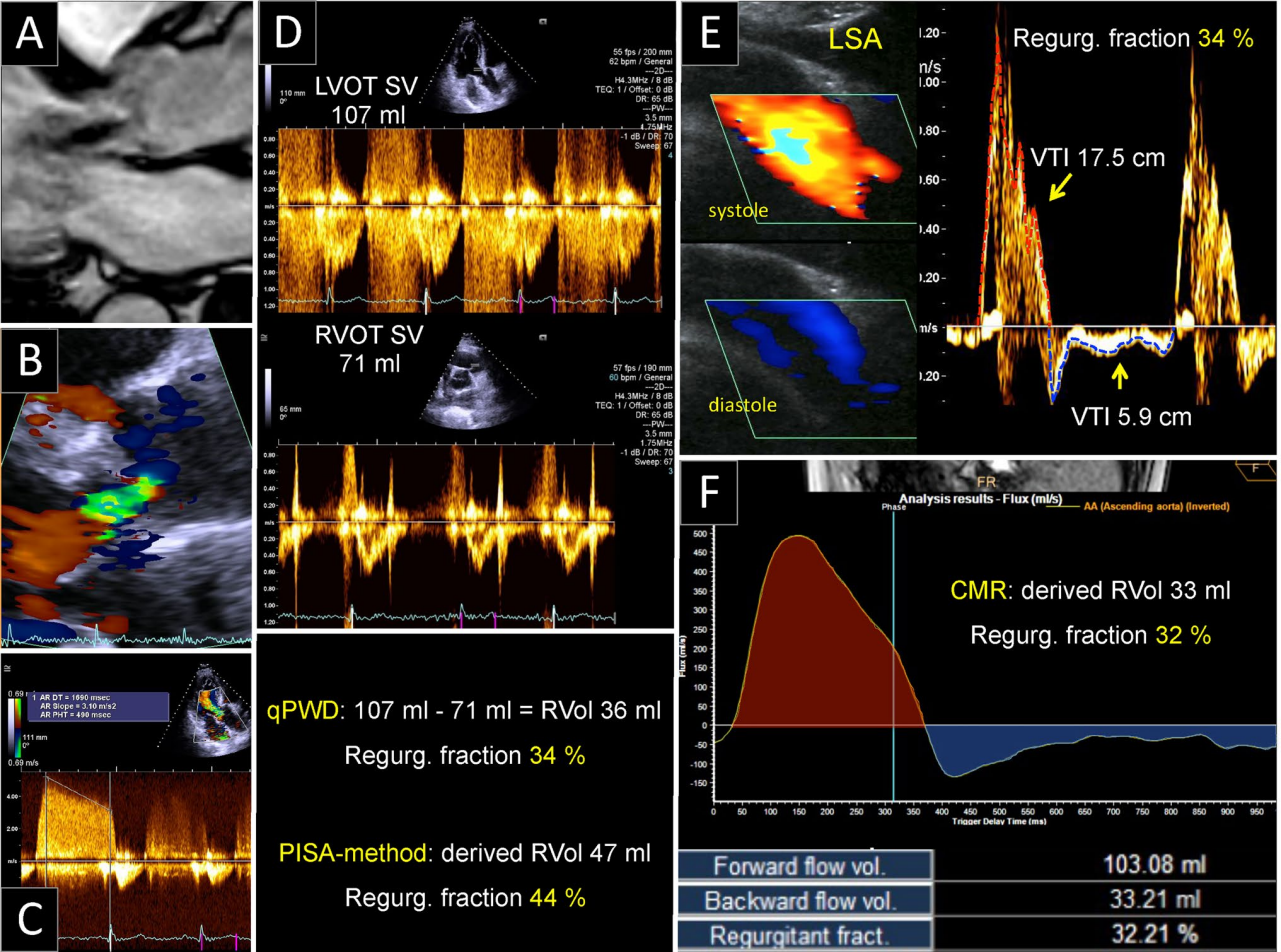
Example of a case with moderate aortic regurgitation (AR). A, B AR-jet in CMR and TTE 3-chamber views. C Continuous-wave Doppler velocity contour of the regurgitant jet depicting a pressure half time of approx. 500 ms. D Quantitative pulse-wave Doppler (qPWD) at the level of the left ventricle (LVOT) and right ventricle outflow tract (RVOT). E Left subclavian artery (LSA) Doppler method. Color Doppler and LSA velocity contours in systole (red) and diastole (blue) are depicted. The correspondence forward and backward VTI are traced from modal velocity. F CMR Q-flow derived regurgitant fraction. *CMR* cardiovascular magnetic resonance, *TTE* transthoracic echocardiography, *VTI* velocity time integral, *RVol* regurgitant volume, *SV* stroke volume
